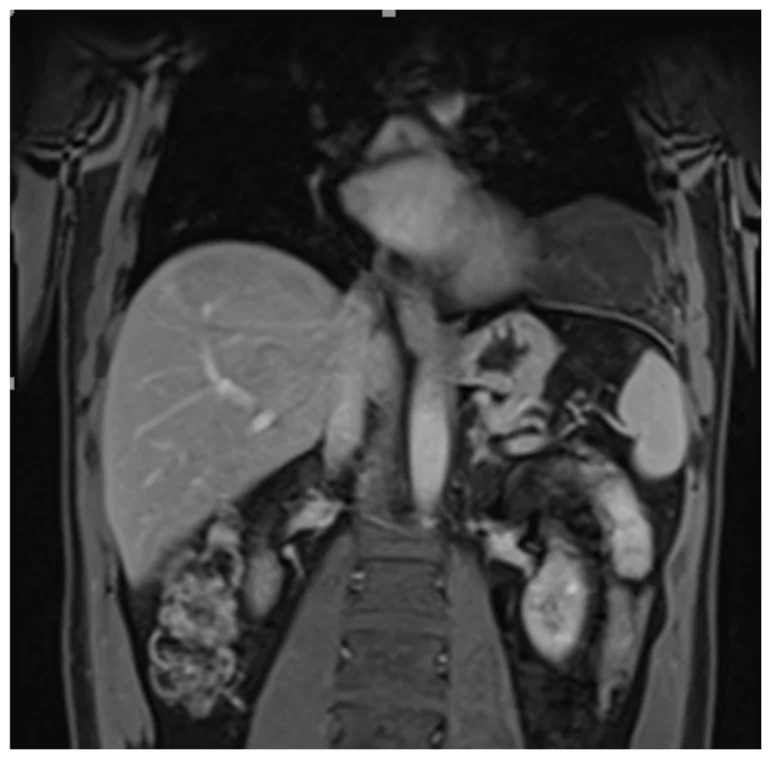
Coronal T2-weighted magnetic resonance imaging (MRI) view confirming a multiloculated, septated cystic lesion (13×9×6 cm) with hyperintense fluid content at the left supradiaphragmatic level.
